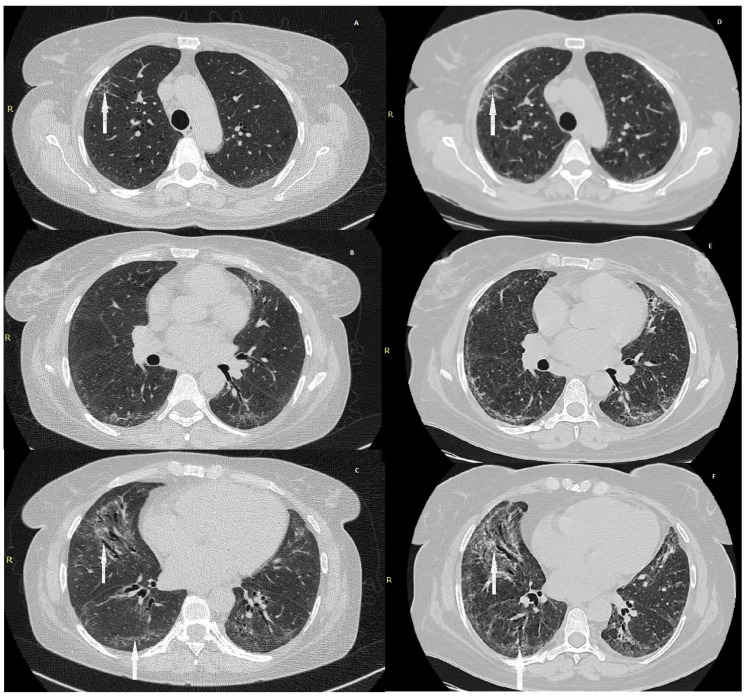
(A–C): High-resolution computed tomography (HRCT) at initial presentation. Subpleural reticulation, interlobular septal thickening, and early traction bronchiectasis are visible, consistent with fibrotic interstitial lung disease. Arrows indicate areas of subpleural reticulation, septal thickening, and early traction bronchiectasis. (D–F): Follow-up HRCT showing progression of fibrotic remodelling, with increased reticulation and architectural distortion. Arrows indicate progression of traction bronchiectasis and fibrotic thickening.
